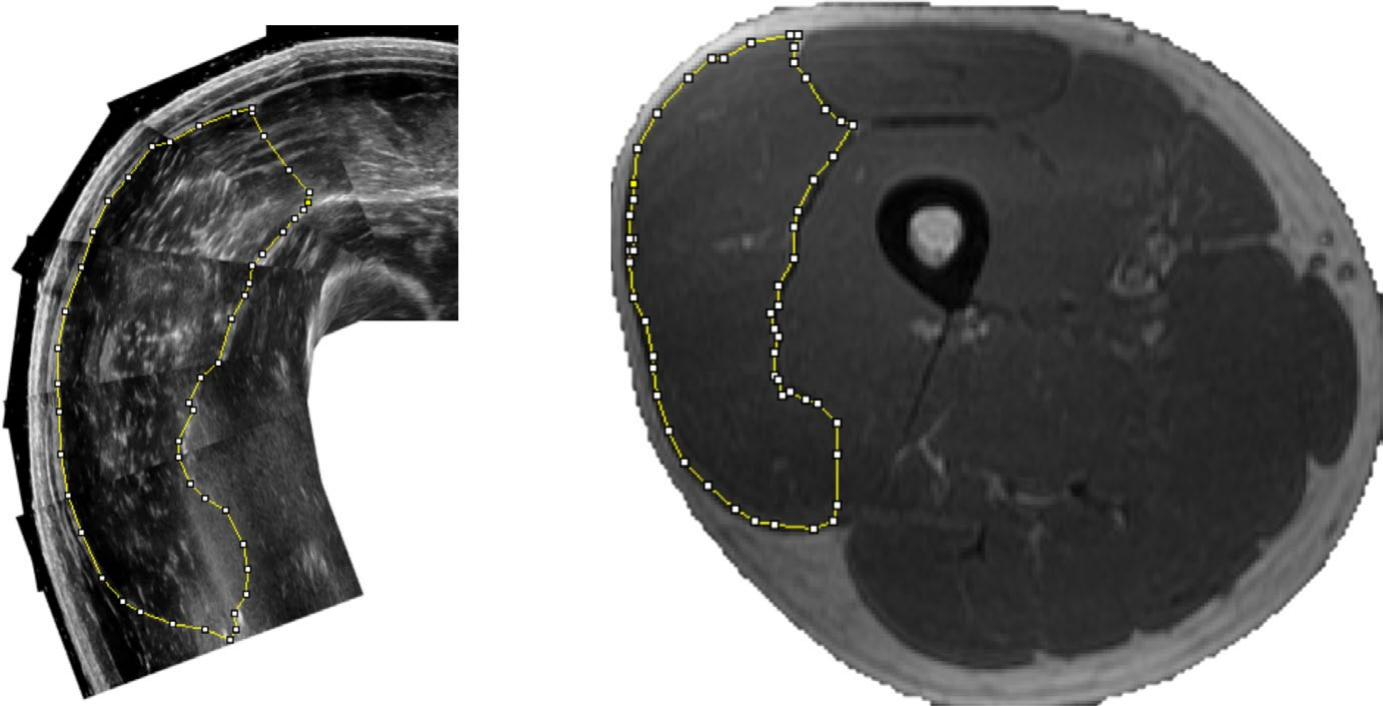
Representatives images of *vastus lateralis* CSA (VLCSA) obtained from (a) B‐mode ultrasonography, and (b) Magnetic resonance. VLCSA was manually outlined in both images using open‐source (ImageJ) software by the investigators in a randomized and blinded manner
